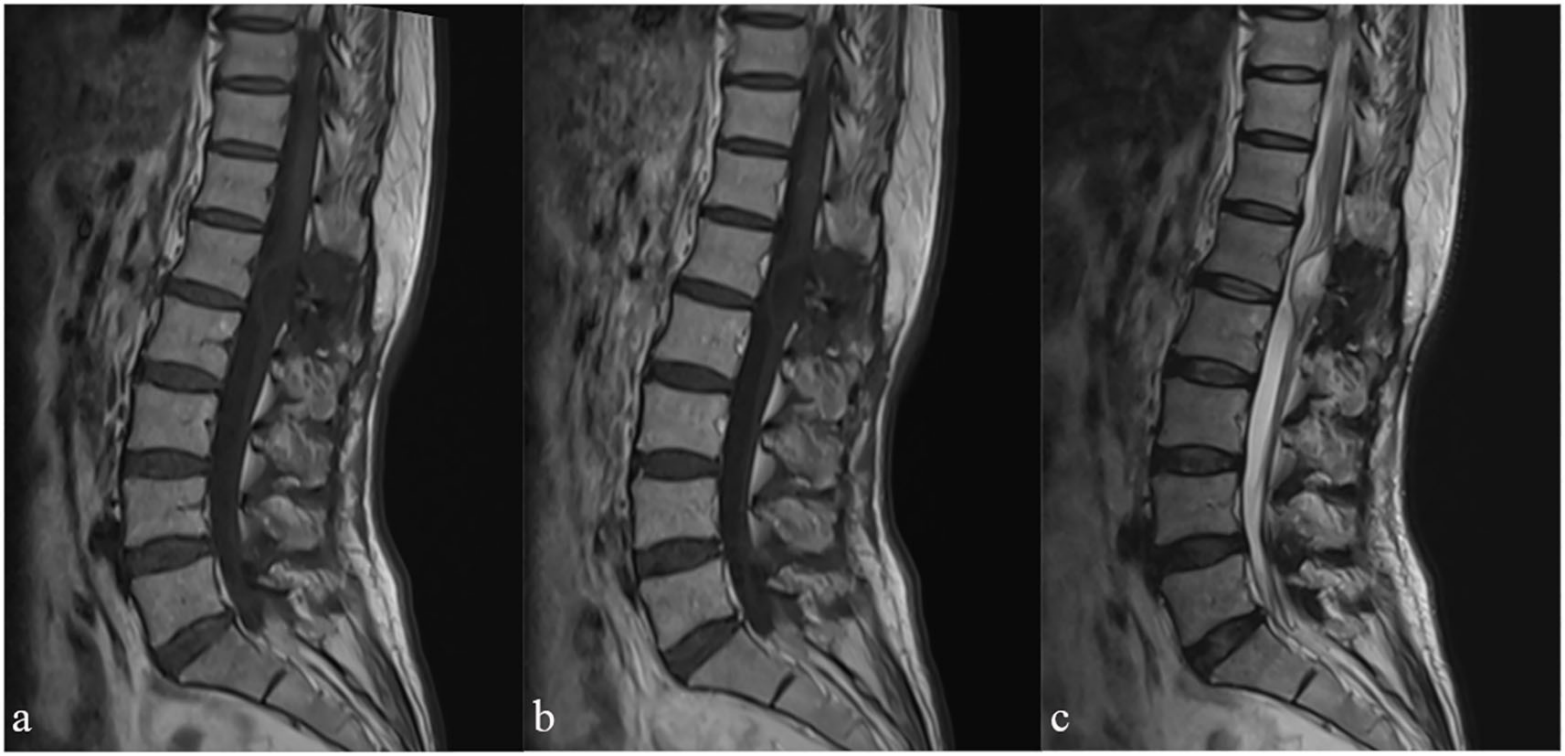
Follow-up magnetic resonance imaging of the lumbar spine, sagittal views: sagittal T1WI (a) T1WI+C (b) T2WI (c).
